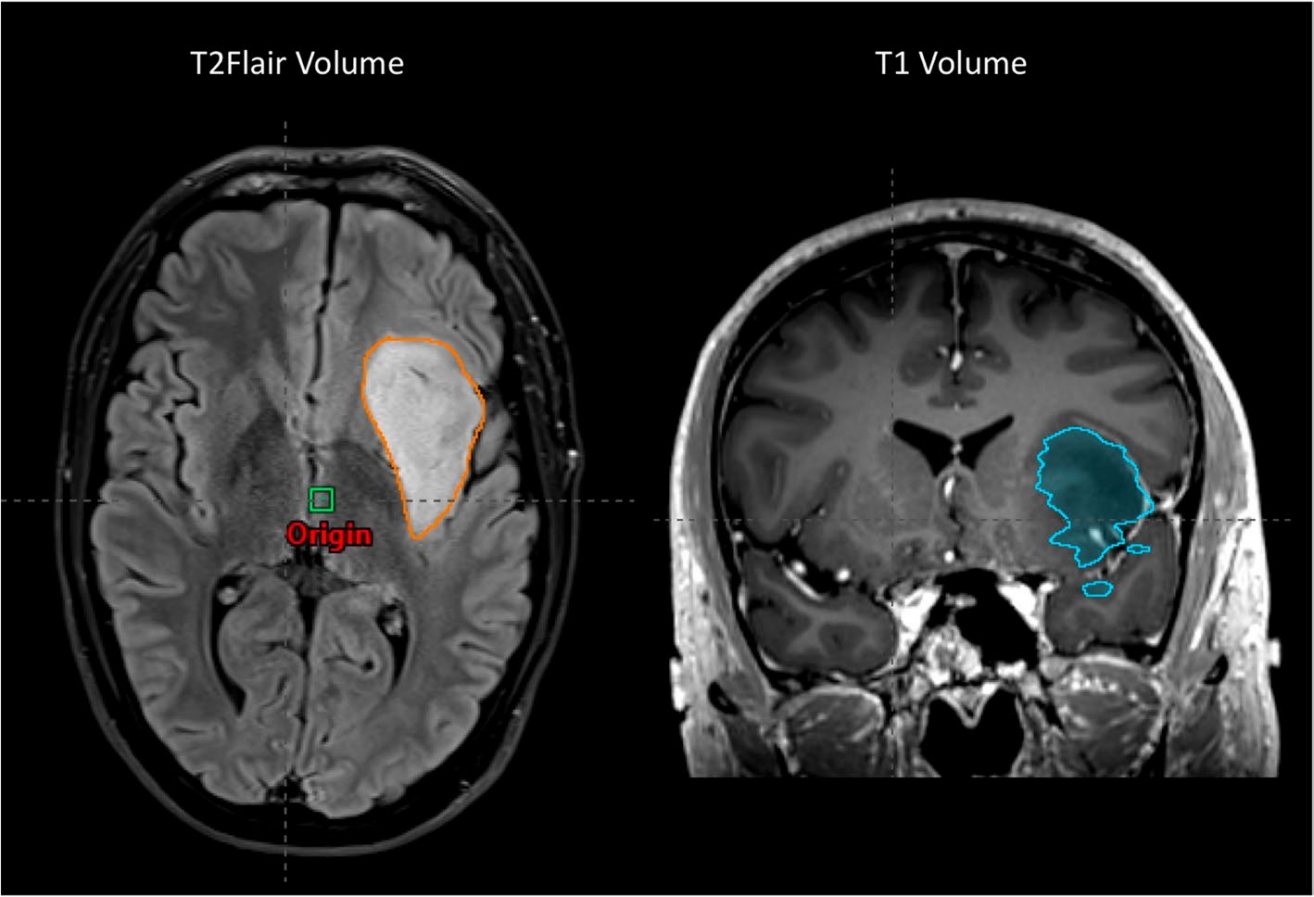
Example of T2Flair and T1 MRI Tumour Volume measurement
